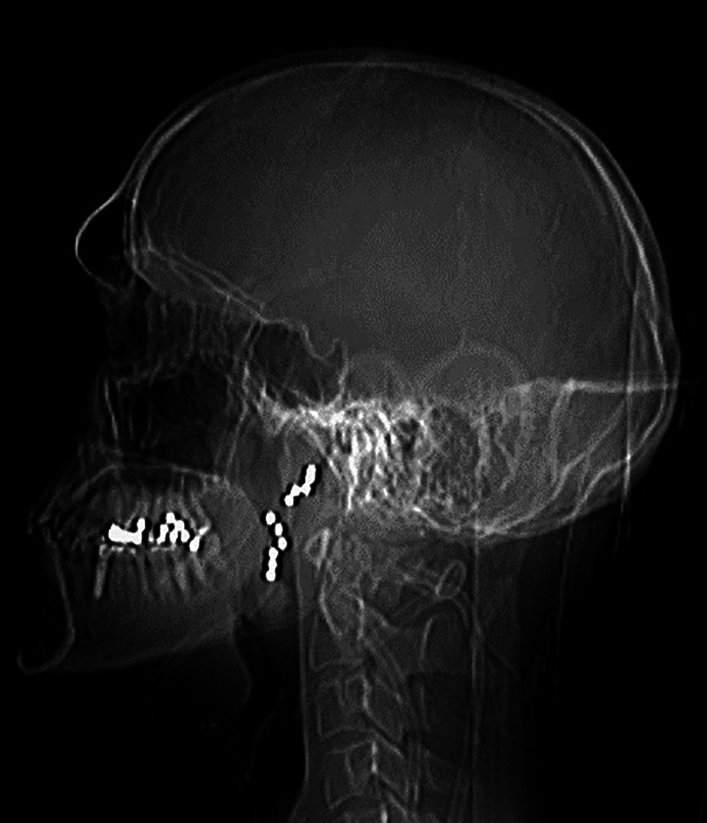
X-ray head after suicide attempt with a shotgun showing multiple shotgun pellets
